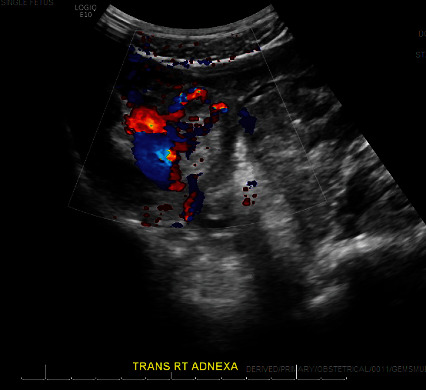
Transvaginal ultrasound of right adnexa. RT: right; TRANS: transverse.
